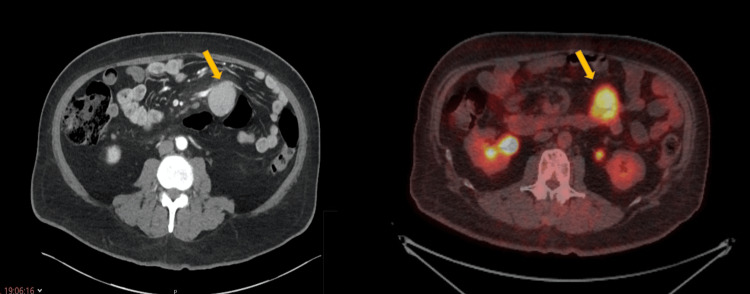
Abdominal mass on work-up, proven to represent UCD. This case had a concurrent biopsy-proven diagnosis of pancreatic cancer. On the left, a CT scan demonstrates a mass (yellow arrow) lying within the pancreatico-duodenal groove. On the right, an FDG PET scan shows intense activity of the mass (yellow arrow), while the primary mass in the head of the pancreas showed no avidity. UCD: Unicentric Castleman disease; FDG PET: Fluorodeoxyglucose positron emission tomography.
